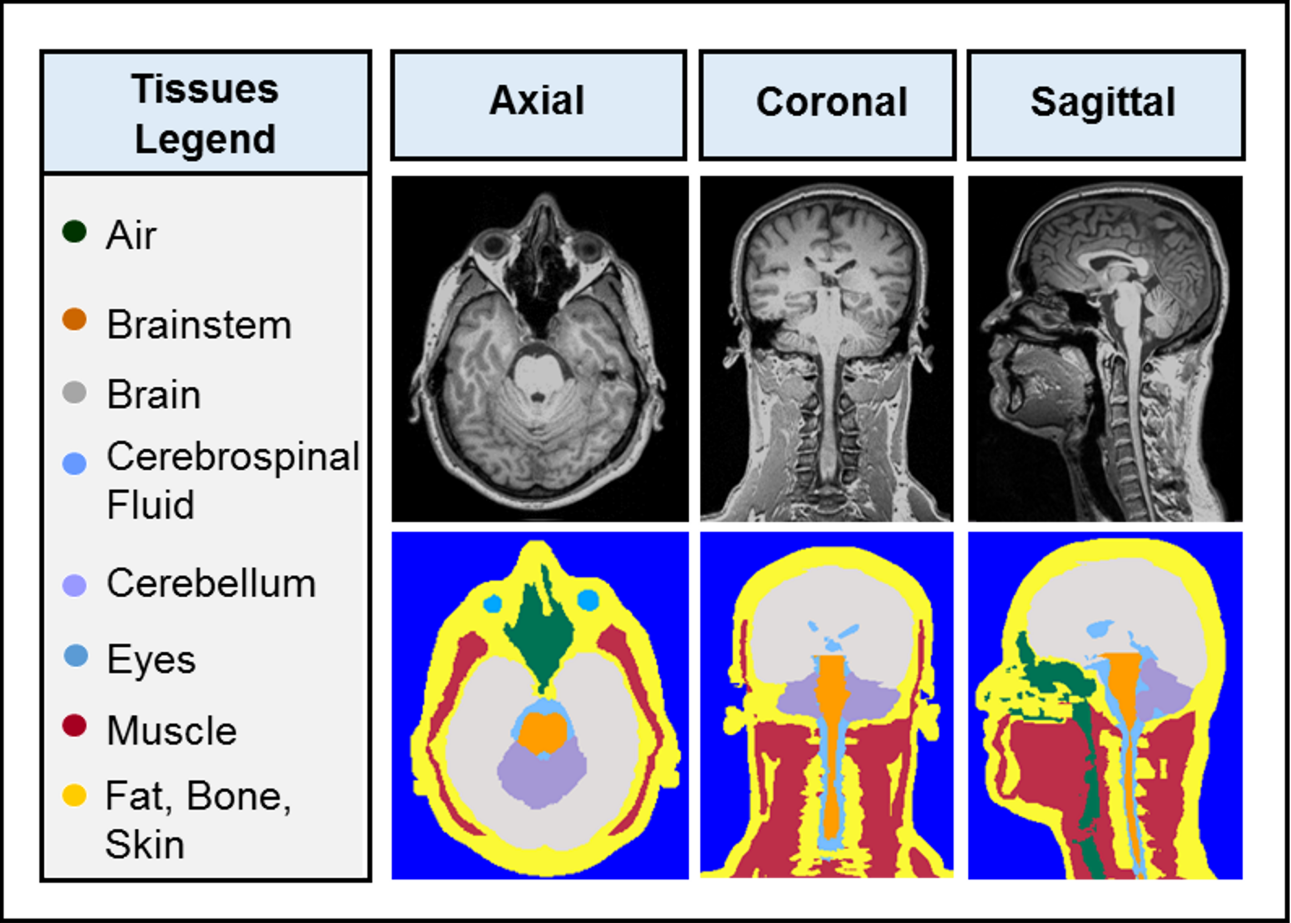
Medical data acquisition and segmentation. A 3T MRI scan with 1.0x1.0x1.0mm^3^ resolution was segmented and divided into eight individual tissues. Each segmented tissue is listed with the corresponding tissue segmentation color within the tissue legend. The pictured MRI dataset and segmented tissues are shown in the mid axial, coronal and sagittal views. Table 1 lists the physiological tissues that were used to classify the tissues in the legend.
